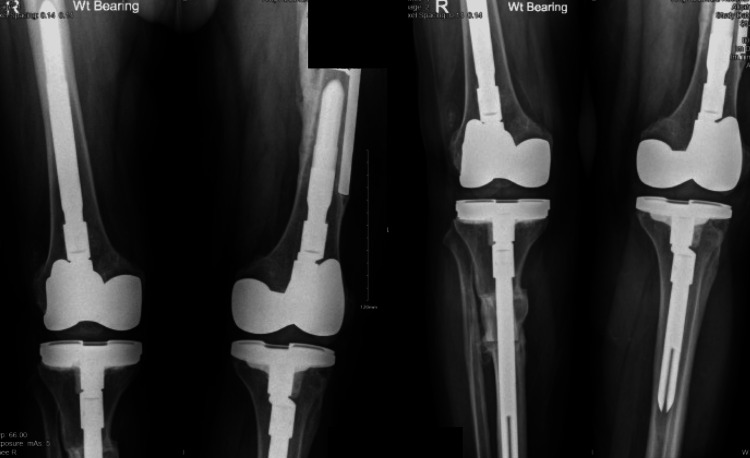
Radiograph showing follow-up one-year mark
